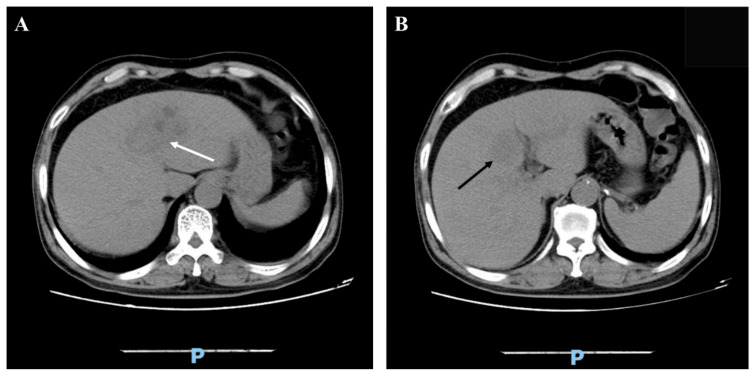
Abdominal computed tomography scan. A: A new abscess had developed in segment II (white arrow). B: The existing abscess in segment IV had decreased in size (black arrow).
